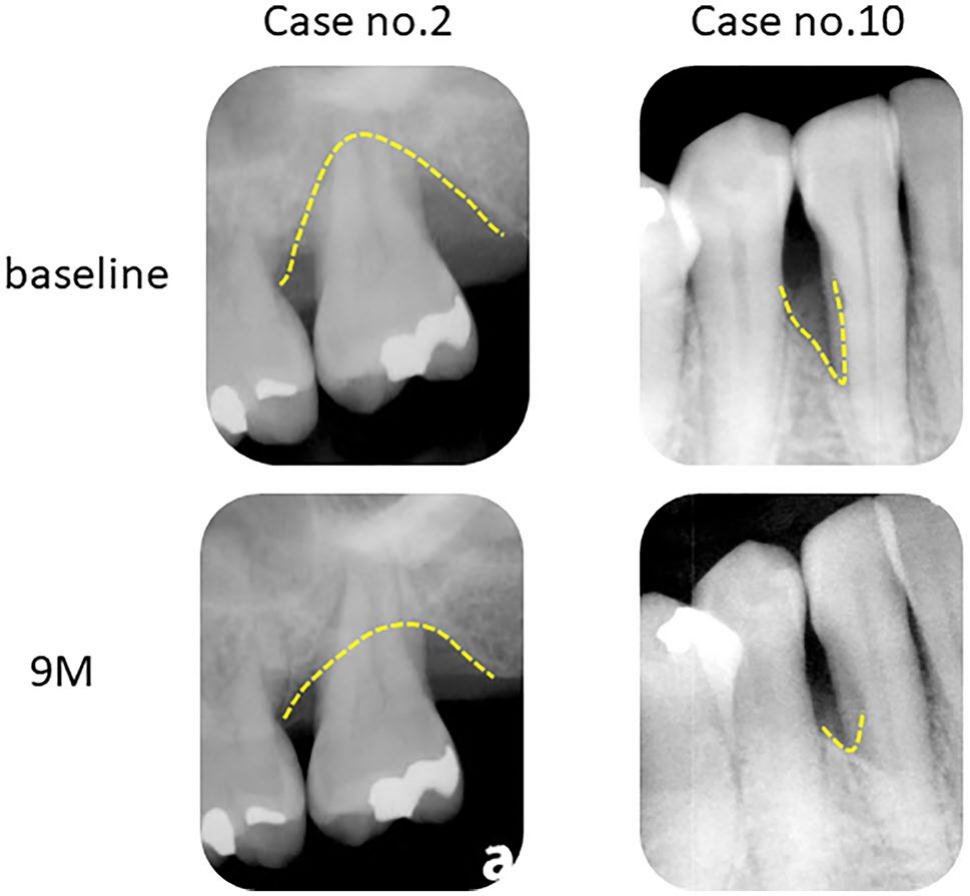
Outcome of adipose tissue-derived multi-lineage progenitor cell (ADMPC) transplantation by dental radiographs. Radiographic outcomes of ADMPC-transplanted individuals. Case no. 2 was a 51-year-old woman. Case no. 10 was a 58-year-old woman. Dotted lines indicate the remaining alveolar bone crest or the bottom of the bone defect. The radiographs clearly show that the bone defect was filled with newly generated alveolar bone at 9 months after transplantation.
